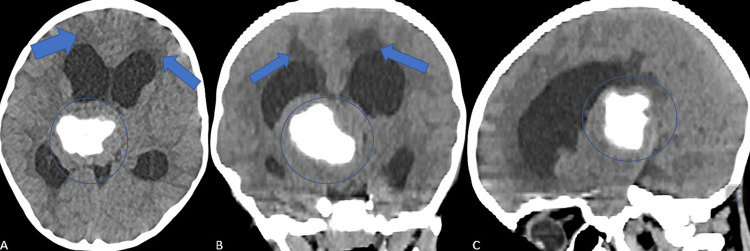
Preoperative non-contrast-enhanced CT scan of a supratentorial ependymoma. A: Axial scan showing that triventricular hydrocephalus caused by the mass lesion was observed. Hypodensities secondary to transependymal CSF transmission were observed in bilateral periventricular white matter (blue arrows). Intralesional hyperdensities were also observed (blue circle). B: Coronal scan showing that from the superior part of the third ventricle, a mass lesion extending to the fourth ventricle, particularly the right side. The interventricular septum cannot be distinguished, the total size is approximately 4.5 cm, and its solid components are isodense with the cortex. The lesion also showed central calcified portions (blue circle). Transependymal CSF transmission was observed adjacent to the superior borders of the lateral ventricles (blue arrows). C: Sagittal scan showing a centrally calcified mass lesion (blue circle), compressing the lateral ventricles. The differential diagnosis should consider ependymoma, central neurocytoma, and pineal region tumors.
